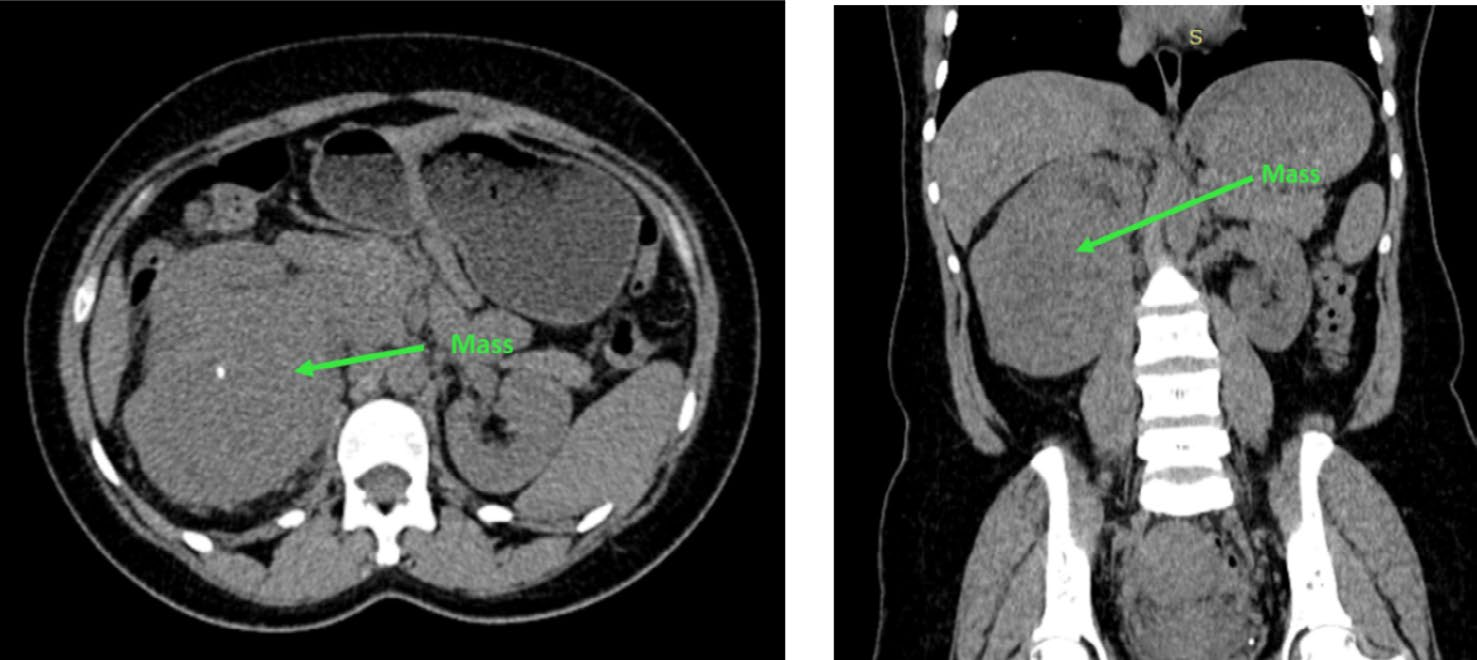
Pre‐contrasted axial and coronal CT scans of the abdomen showing a soft tissue mass in the right kidney with a calcified foci.
